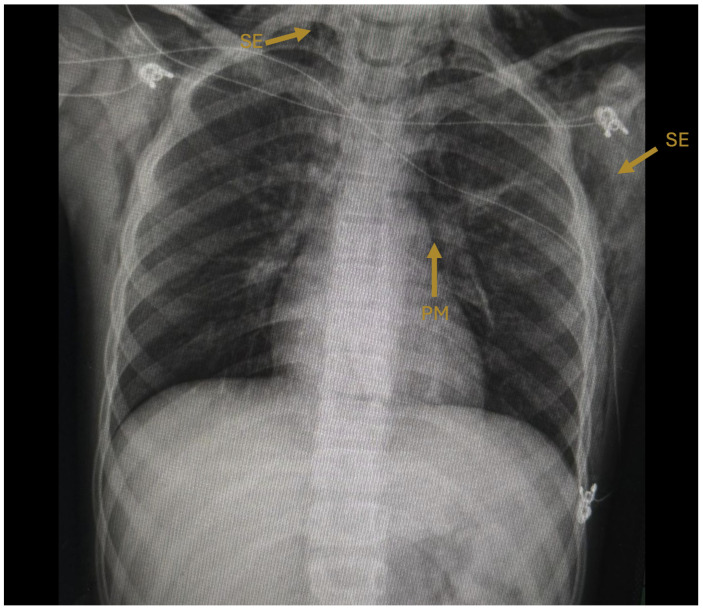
Initial posteroanterior chest radiograph (hospital day 1). Arrows indicate pneumomediastinum (PM), visible as a radiolucent halo outlining the cardiac silhouette, and subcutaneous emphysema (SE) dissecting through the cervical and pectoral soft tissues bilaterally. The trachea is midline and pulmonary parenchyma appears without consolidation.
